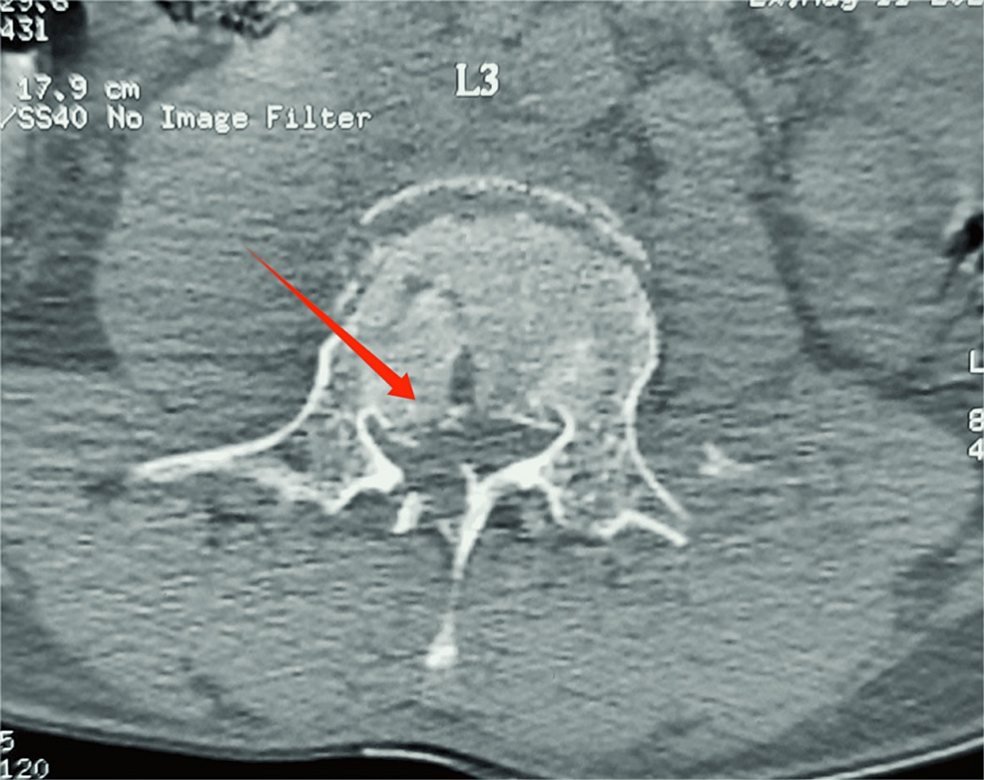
CT showing L3 burst fracture with retropulsion of fragment CT, computed tomography
